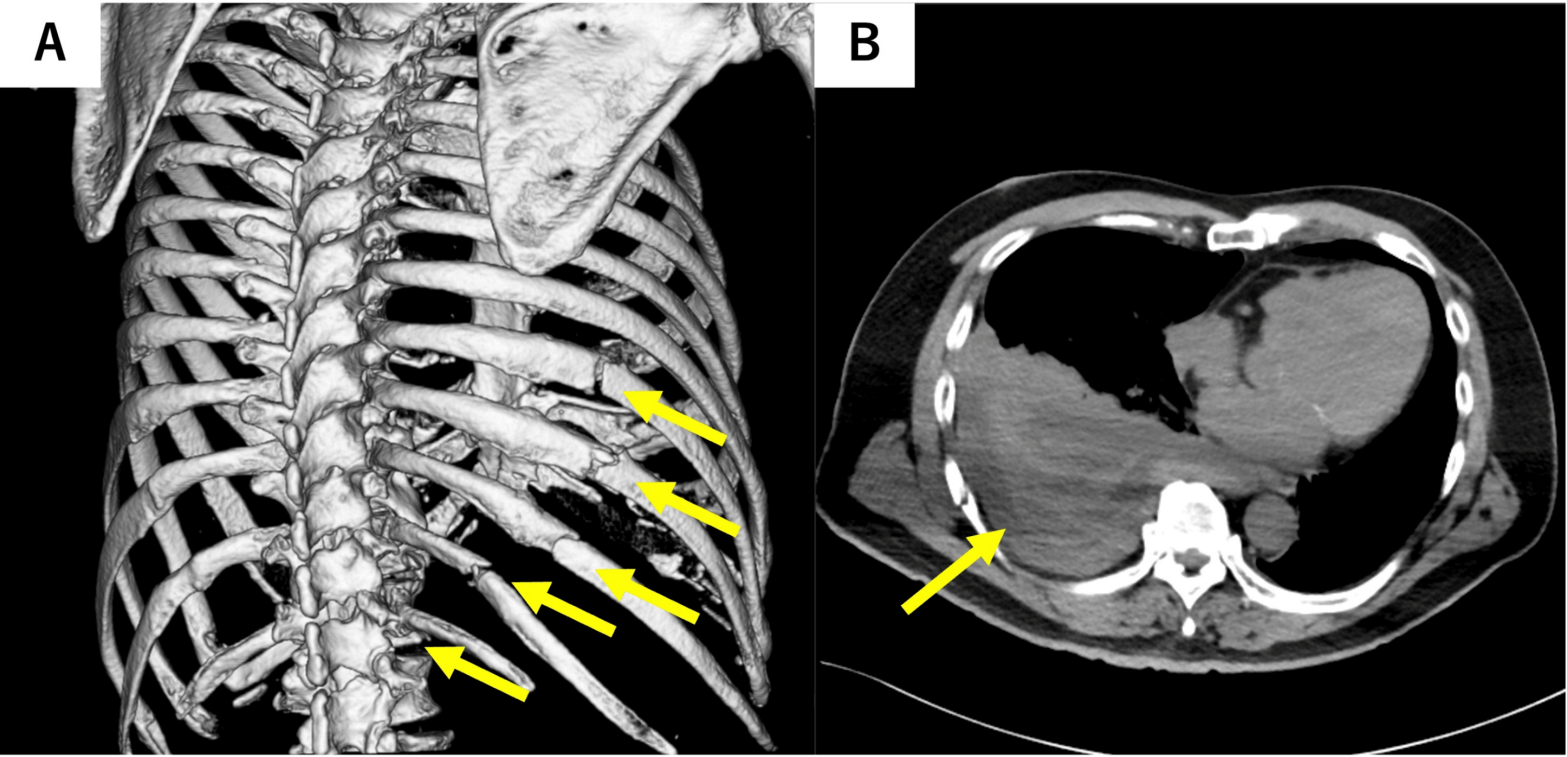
Rib fractures and hemothorax. (A) Three-dimensional CT bone reconstruction showing multiple healing fractures of the right 8th-12th ribs (arrows). (B) Axial non-contrast CT showing a large right pleural effusion compatible with hemothorax (arrow).
